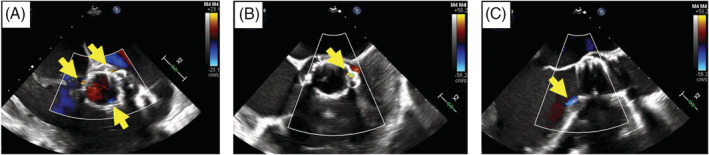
Intraoperative TEE images of patient 1. A, Multiple jets of PVR (yellow arrows; Supplemental Video 1). B, PVR after ViV implantation (yellow arrow; Supplemental Video 2). C, Post‐procedure trivial PVR (yellow arrow; Supplemental Video 3). PVR, paravalvular regurgitation; TEE, transesophageal echocardiogram; ViV, valve‐in‐valve
